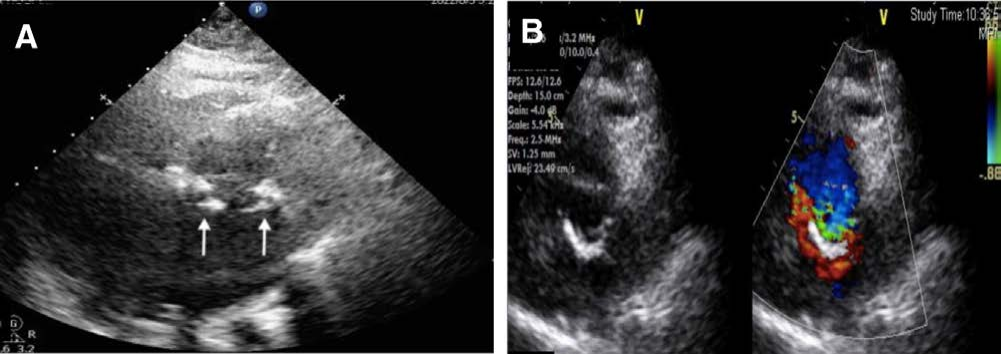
(A) Nonstandard apical 4-chamber views exhibited echoes consistent with a residual broken suture ring or endocardial appearance in the mitral position. (B) On the horizontal short-axis view of the mitral position, robust echoes resembling the prosthetic valve ring were still observed. However, A and B introduced some confusion and conflicting ultrasound information due to the limitations of TTE. TTE = transthoracic echocardiography.
